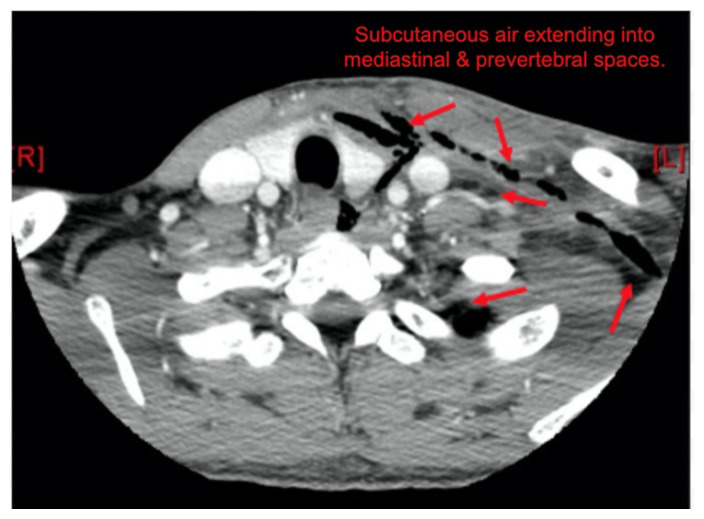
Axial CT Video Link: https://youtu.be/aqTOSnGU0vg
